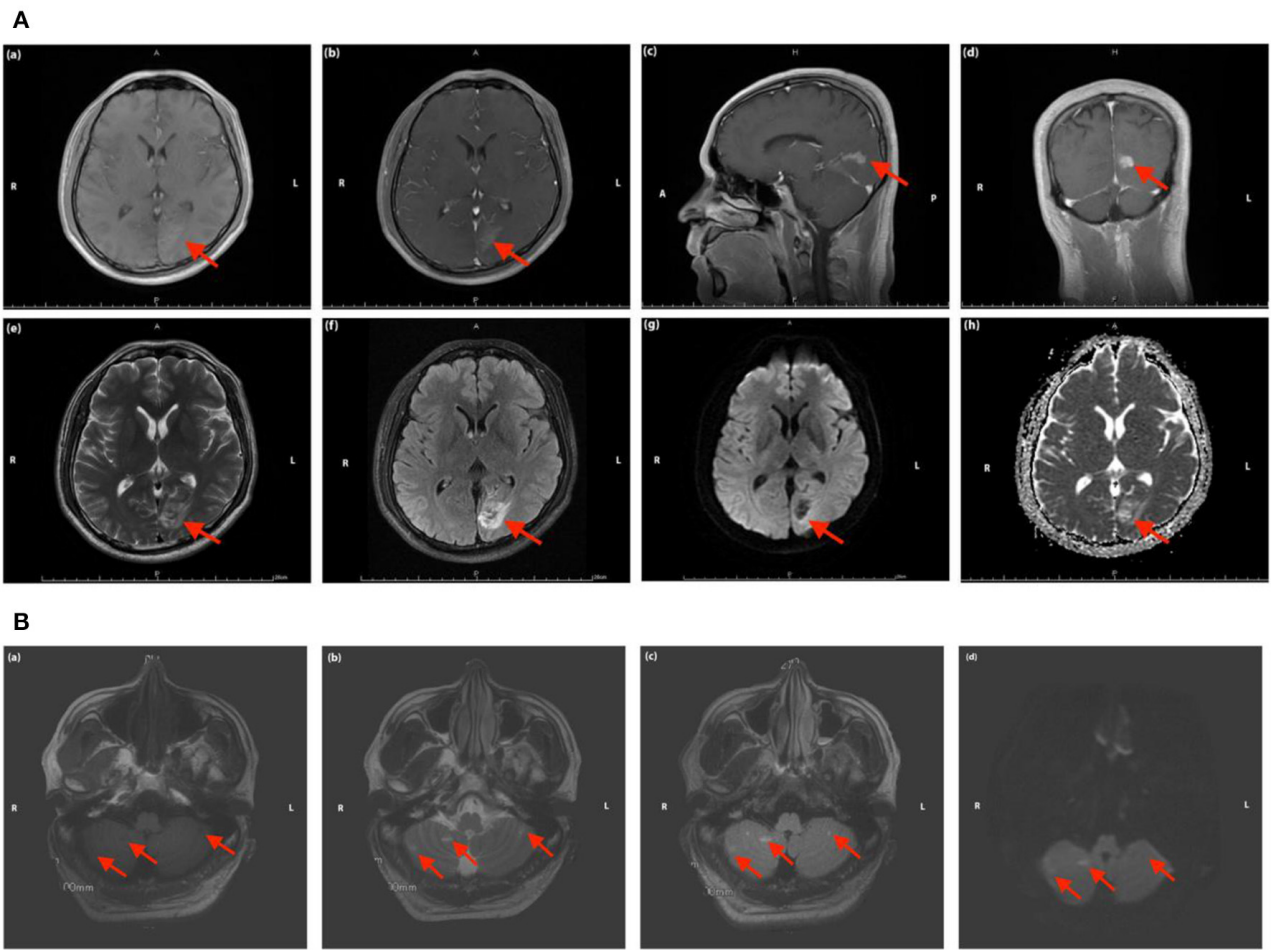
(A) Magnetic resonance images of the head in Case 2. The red arrow indicates the focal ischemic infarction in left occipital lobe [(a) T1 sequence; (b–d) T1 sequence with contrast-enhancing; (e) T2 sequence; (f) T2 FLAIR sequence; (g) DWI sequence; (h) ADC sequence]. (B) Magnetic resonance images of the head in Case 2. The red arrow indicates the focal ischemic infarction in cerebellum [(a) T1 sequence; (b) T2 sequence; (c) T2 FLAIR sequence; (d) DWI sequence].
